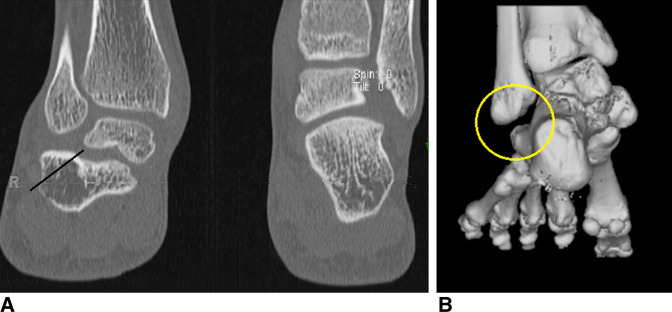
Lateral Impingement. A and B, Preoperative CT scan of a patient with a history of congenital clubfoot deformity and report of lateral hindfoot pain. The CT scan demonstrates subfibular impingement of the lateral calcaneus. Treatment included a lateral calcaneal wall exostectomy and débridement of the subfibular region. The black line represents an approximation of the bone removal.
